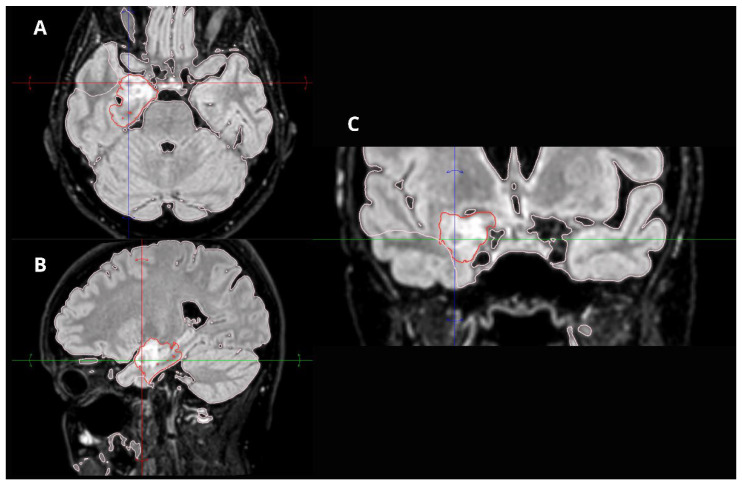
Preoperative FLAIR MRI of the patient’s dysembryoplastic neuroepithelial tumor (DNET) in the right temporal lobe. (A) Axial FLAIR scan showing a clearly delineated lesion with central hypointensity, suggestive of possible necrosis. (B) Sagittal FLAIR scan depicting the lesion’s extent and its well-defined borders. (C) Coronal FLAIR scan highlighting the lesion’s relationship to surrounding structures, with central hypointensity consistent with potential necrosis.
